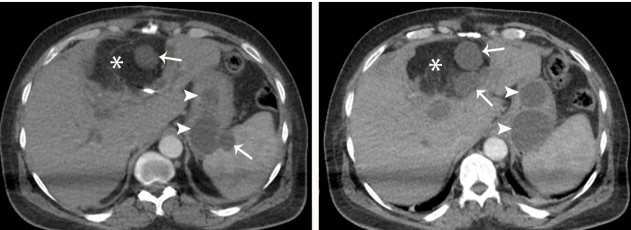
Computed tomography of the abdomen showing multiple cystic lesions in the liver, spleen, and stomach (arrows). Parenchymal loss was observed in the liver due to cystectomy (asterisk). Stomach wall hydatid cysts extend to intracavitary areas (arrowheads).
